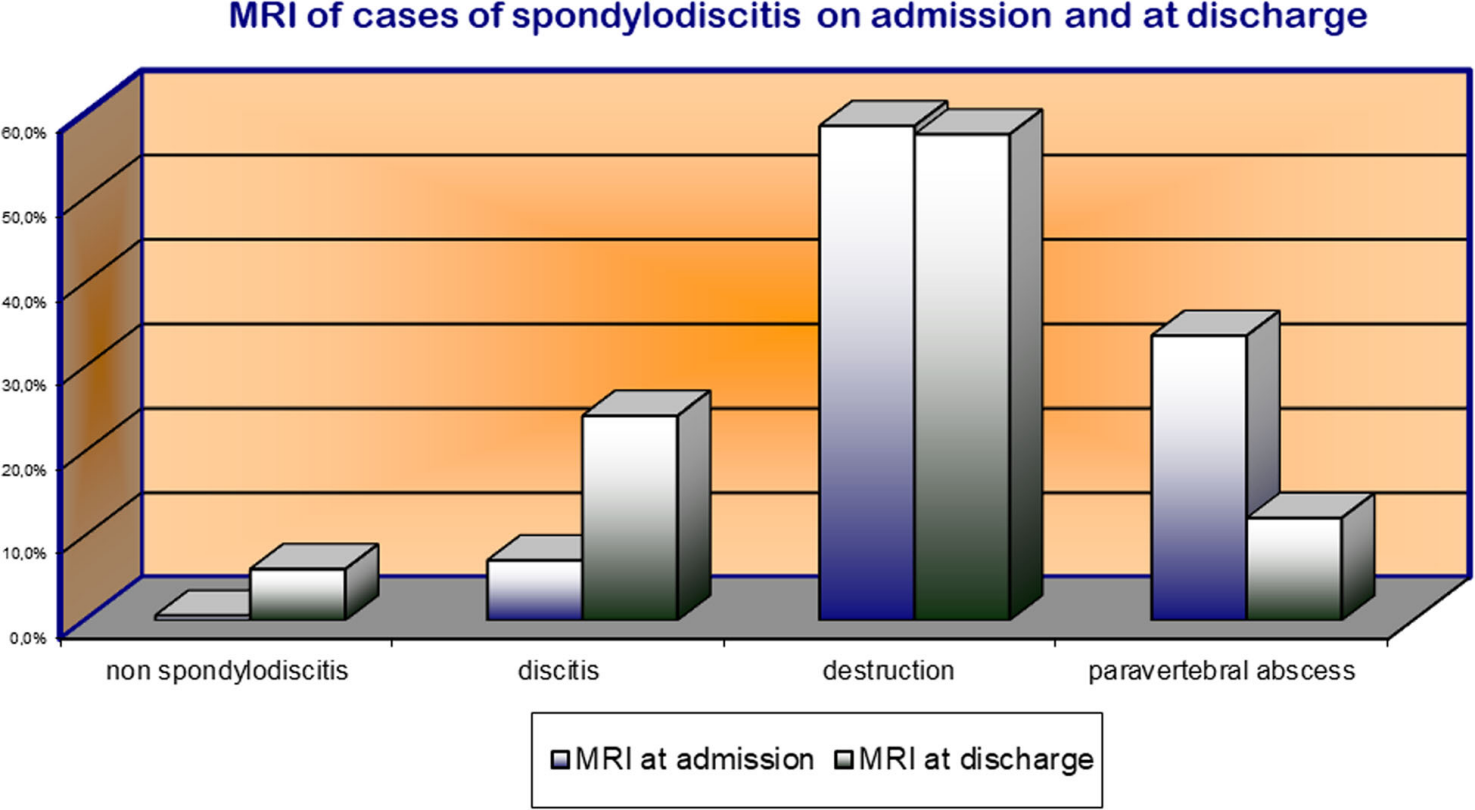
MRI of cases of spondylodiscitis on admission and at discharge
